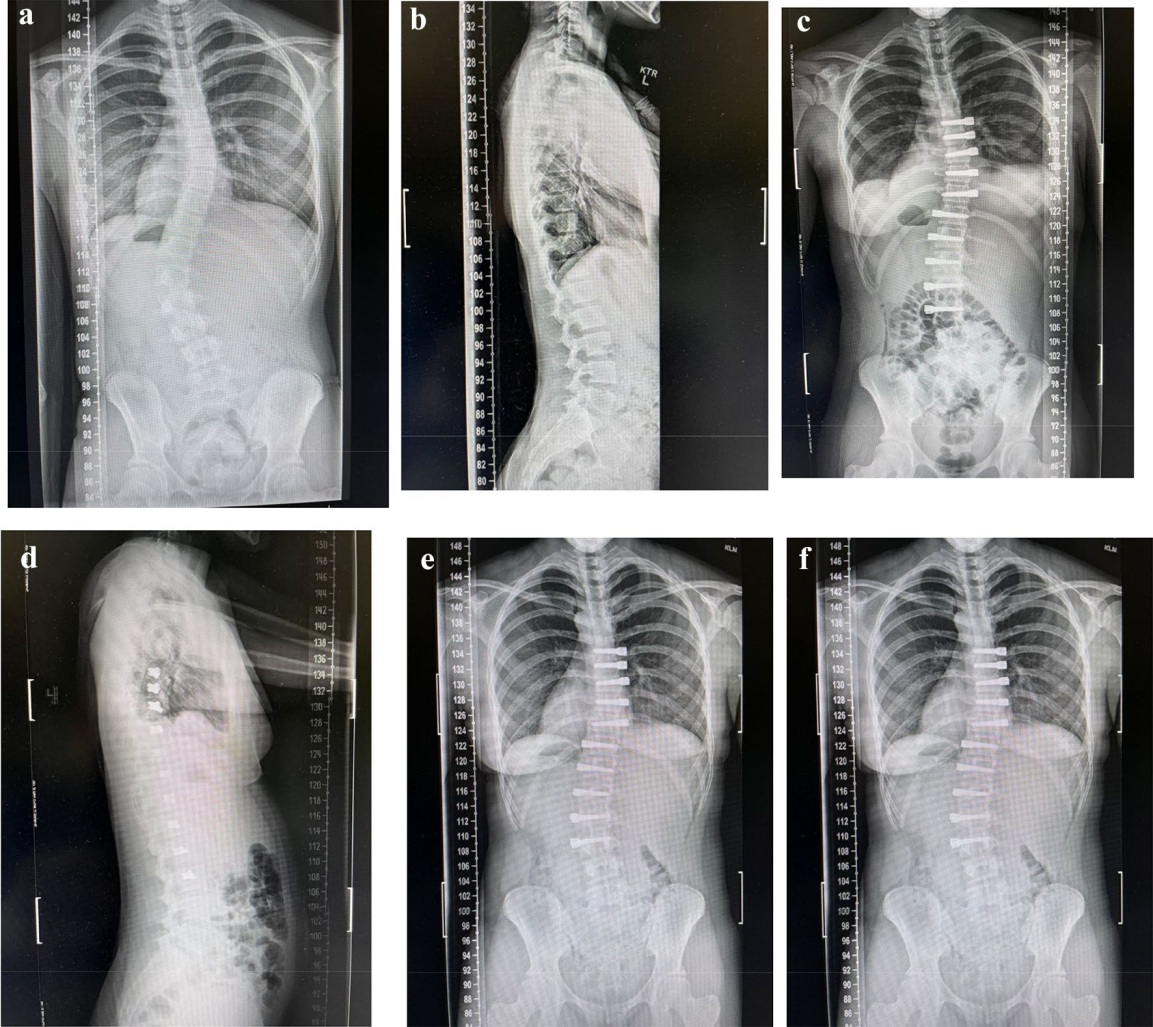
A–F (TIFF images 1–6). A 15 year old skeletally immature female with progressive 40° right thoracic and 48° left thoracolumbar curves (Lenke 6C) at Risser 1 was treated with AVT right T5-T10 and left T11-L3 with initial curve correction to 20° and 14°, respectively, and subsequent additional correction with growth over 2 years to 10° and 17°, respectively, at Risser 4. The post-operative left shoulder elevation resolved over time. [A–B (TIFF images 1–2) Pre-operative posteroanterior and lateral radiographs; C–D (TIFF images 3–4) Postoperative posteroanterior and lateral radiographs; E–F (TIFF images 5-6) Follow-up posteroanterior and lateral radiographs at 2 years]
